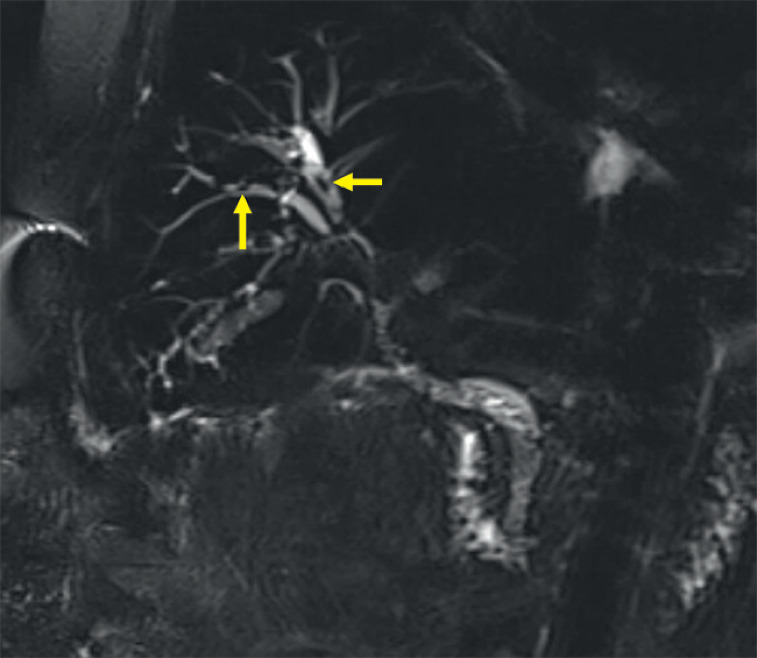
Magnetic resonance imaging revealing filling defects (arrows) within the intrahepatic ducts suggestive of debris or fungal balls.
